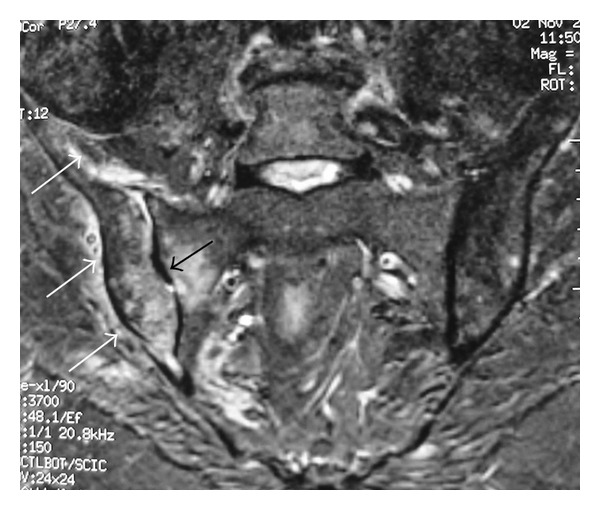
Coronal T2-weighted fat-suppressed MR image of the pelvis shows an increased signal intensity of the right sacroiliac joint (black arrow) and diffuse hyper intensity of the adjacent iliacus and gluteal muscles (white arrows).
